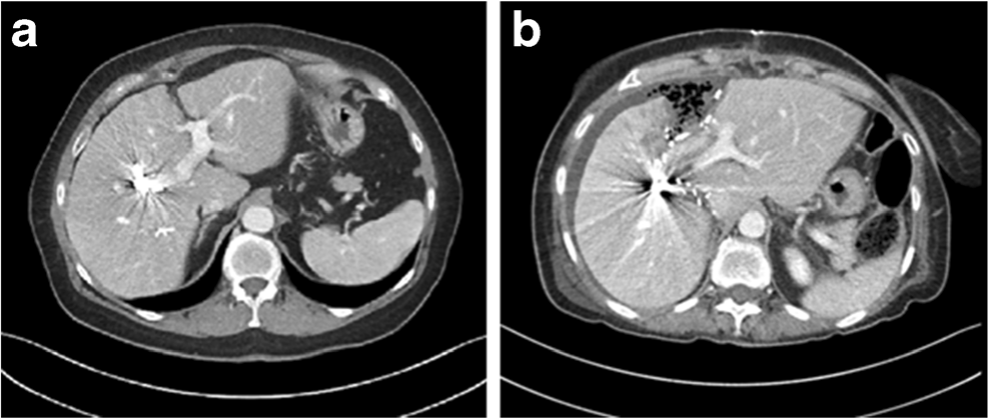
Example of rescue ALPPS after failed portal vein embolization CT after PVE (a) and CT before stage 2 (b) in the same patient
